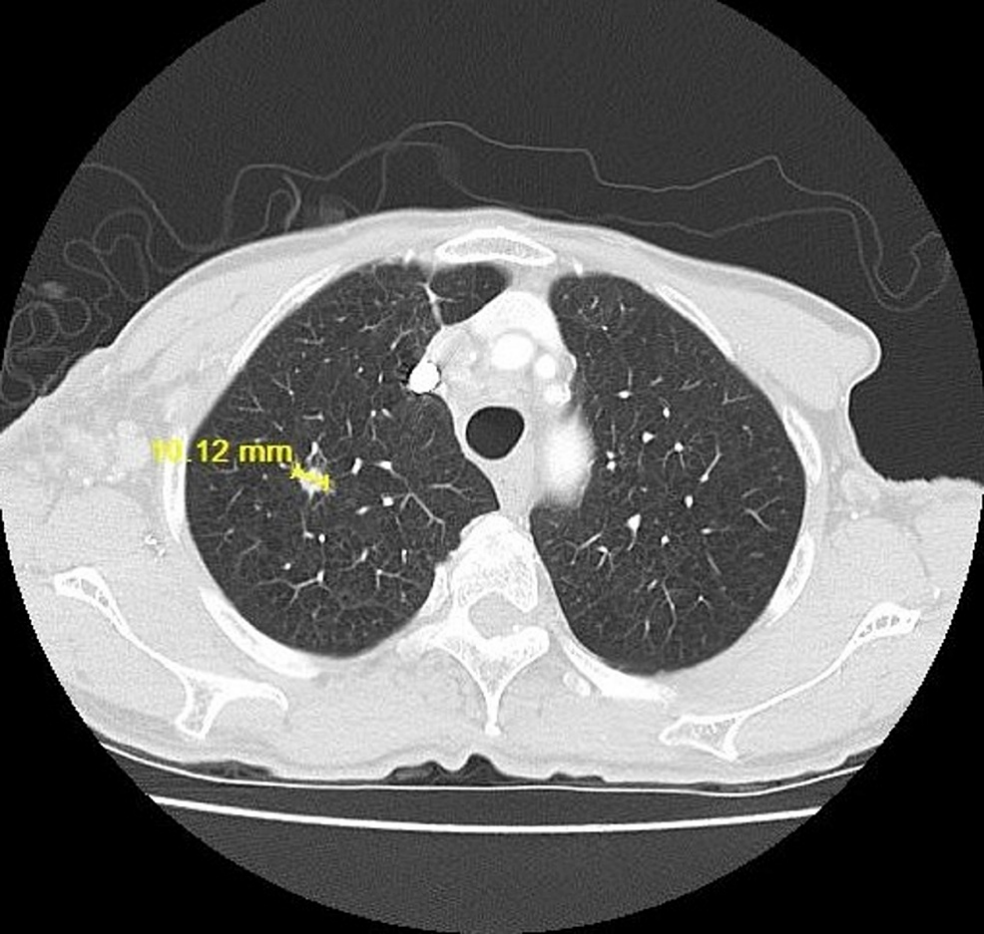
CT chest lung window showing right upper lobe nodule on 06/12/2017
